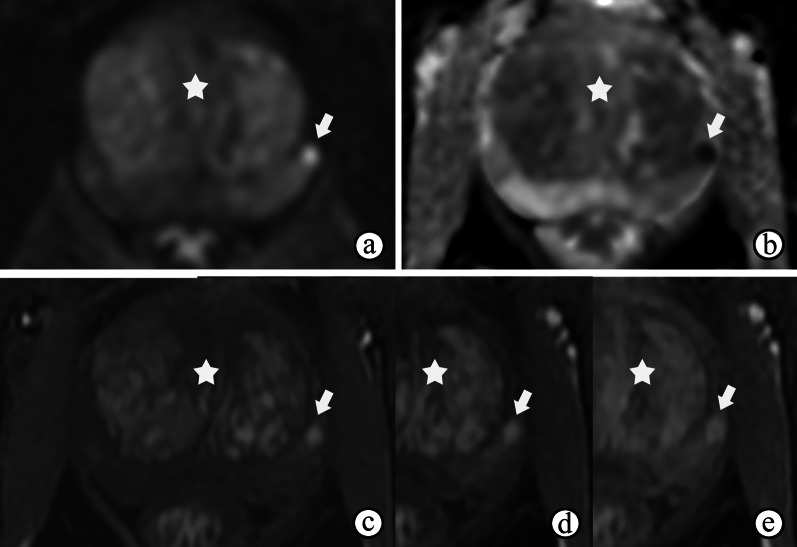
Nonspecific granulomatous prostatitis in a 57-year-old male with dysuria and urinary retention for 1 month and a serum PSA level of 13.22 ng/mL. a, b DWI at a b-value of 1400 s/mm^2^ and ADC maps show a focal nodule with obviously restricted diffusion (arrow) in the left peripheral zone of the prostate. c–e DCE imaging shows early and prolonged enhancement of the nodule (arrow). The appearances of the nodule in a–e result in a PI-RADS score of 4. Note the prostatic hyperplasia in the TZ (☆), which may have caused the symptoms of dysuria and urinary retention
